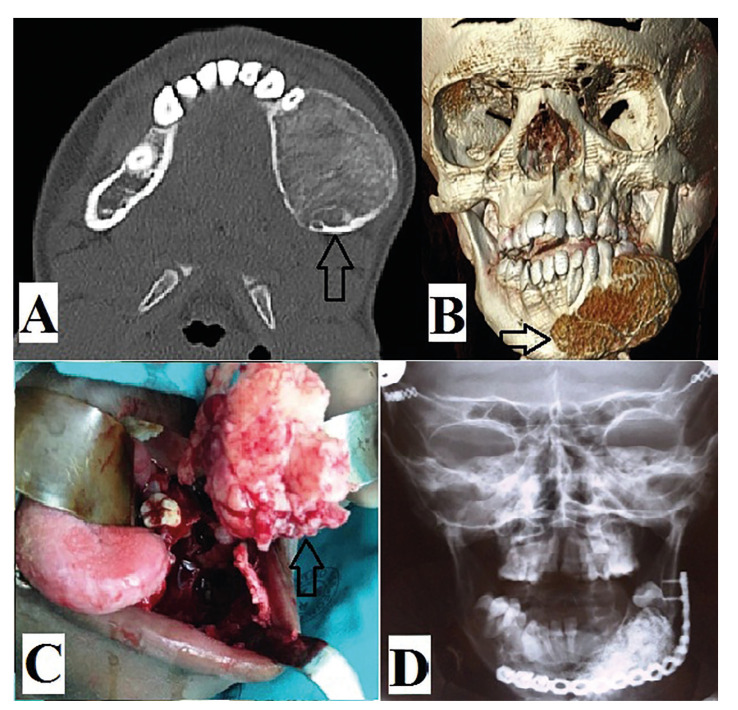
A: Axial CT of JPOF in the posterior mandible showing well-defined mixed density lesion. B: 3D reconstruction of CT showing destruction of mandibular cortex. C: Intra-operative photograph post curettage of lesion. D: Post-operative posterio-anterior radiograph of the mandible following reconstruction of the defect.
